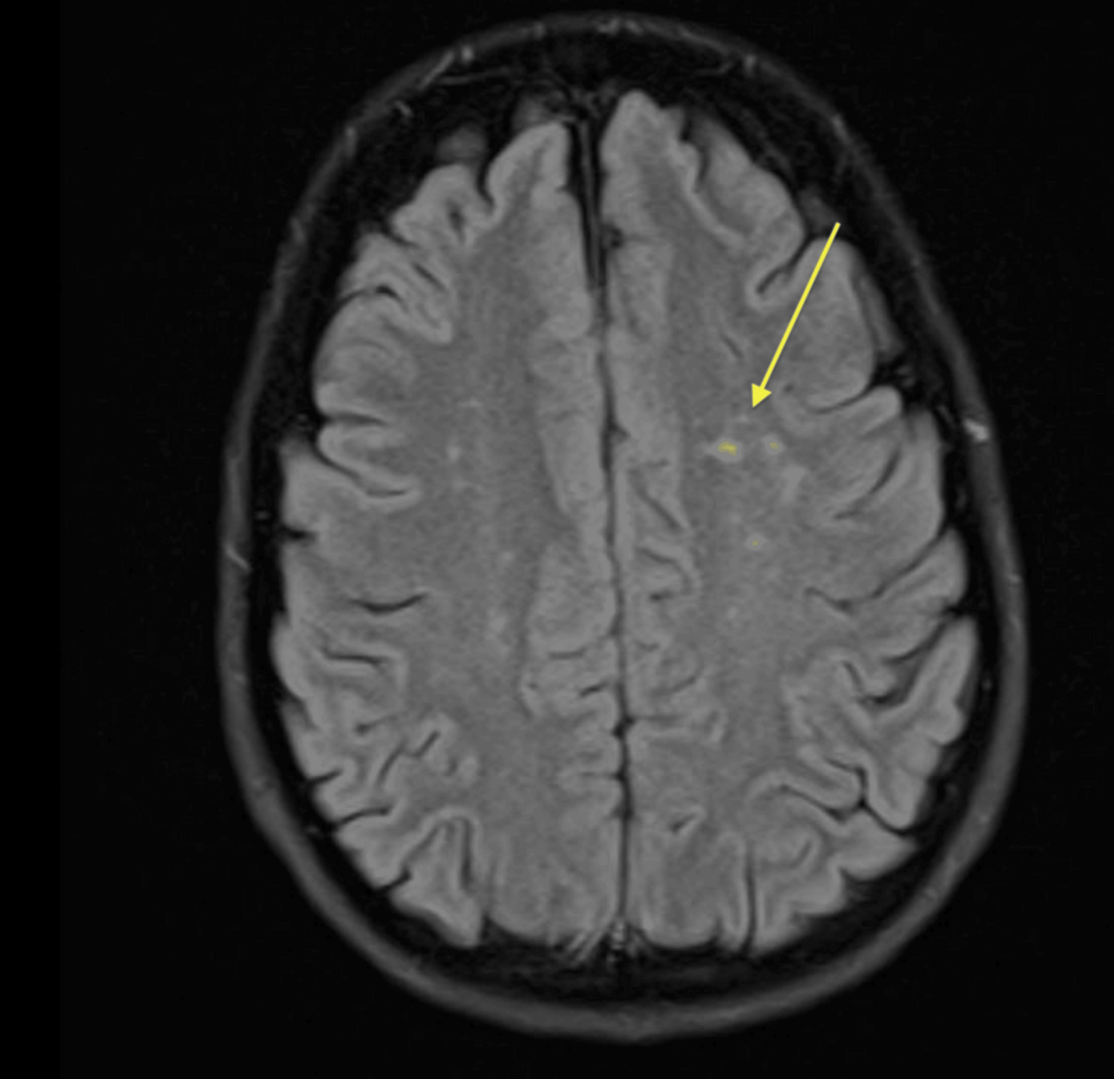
MRI head showing a small focus of restricted diffusion within the right thalamus, which may represent a recent infarct. A small subcortical focus of restricted diffusion is also seen in the left frontal lobe, with a further focus posteriorly in the left parietal lobe (yellow arrow).
